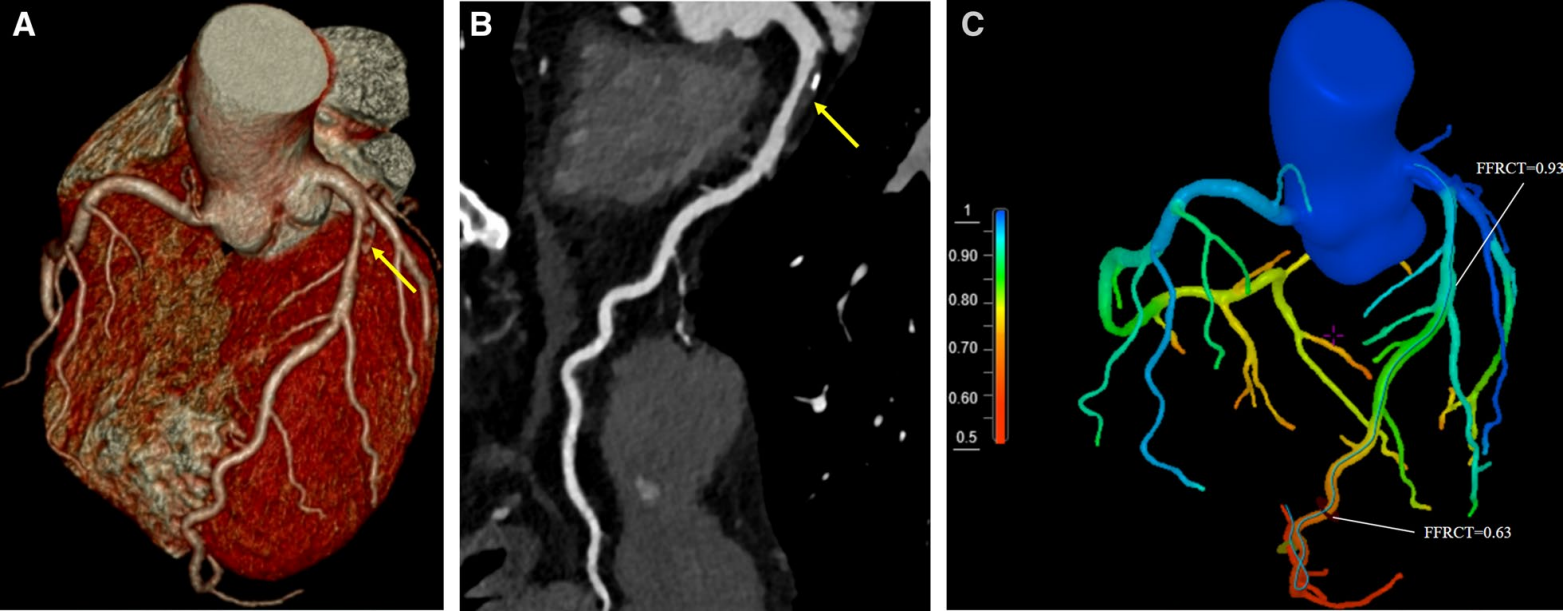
Representative case of patient with MACE. A 58-year-oid male with unstable angina. CCTA showed mixed plaque with moderate stenosis at proximal LAD (yellow arrow in A, B). This lesion was revealed to be hemodynamically insignificant with FFRCT value of 0.93 at proximal LAD, while hemodynamically significant with FFRCT value of 0.63 at distal LAD (C). The patient was treated with medical therapy initially, but 2 months later he suffered a sudden acute anterior myocardial infarction, and underwent emergency revascularization. CCTA, coronary computed tomography angiography; FFRCT, fractional flow reserve derived from computed tomography; MACE, major adverse cardiac events; LAD, left anterior descending
